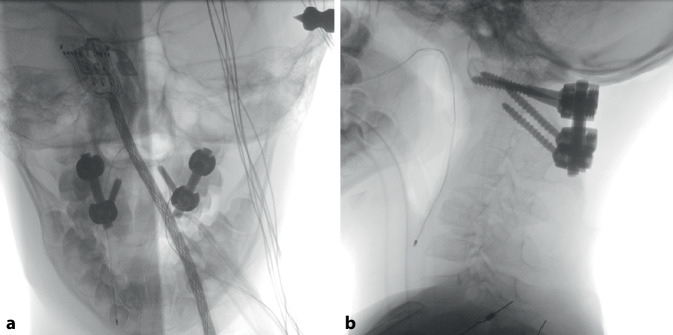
Intraoperative radiographs in lateral (a) and anteroposterior (b) planes after stabilization procedure. a anteroposterior view and b lateral view
